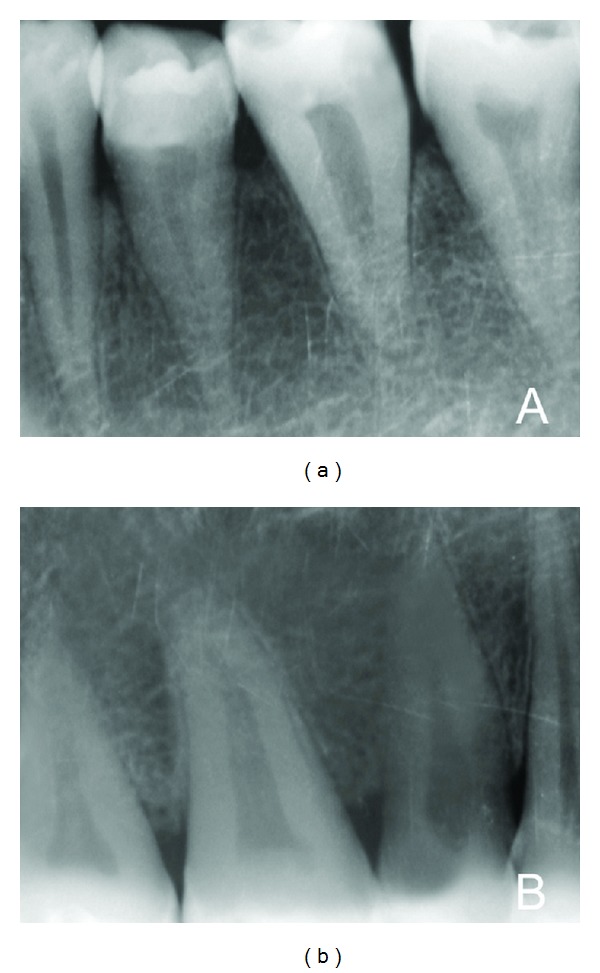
(a) Radiograph shows all the mandibular posterior teeth: contralateral side. (b) Radiograph shows all the right maxillary posterior teeth with single root.
